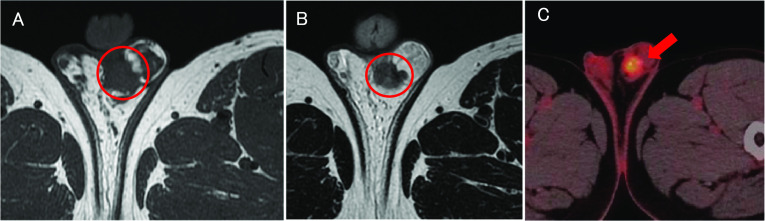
Magnetic resonance imaging (MRI) and Positron emission tomography (PET)–CT findings of the left testis. A MRI T1-weighted imaging revealed a 17 mm tumour with a low signal intensity on the cephalic side of the left testis (red circle). B MRI T2-weighted imaging revealed a 17 mm tumour with a low signal intensity on the cephalic side of the left testis (red circle). C PET–CT revealed a SUV max of 4.4 FDG accumulation, confined to the cephalic side of the left testis (red arrow)
